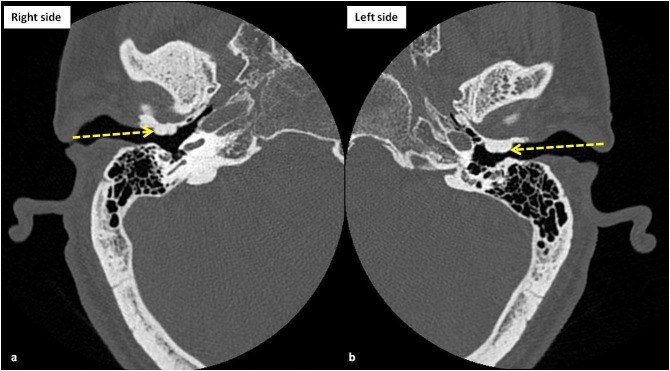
The computerized tomography (CT) scan images (bone windows) of right (a) and left (b) temporal bones at the level of external auditory canals show nearly symmetrical smooth bony outgrowths (yellow arrows), arising from the anterior walls of the external auditory canals, consistent with exostoses. Please note that the exostoses are causing significant narrowing of the external auditory canals leading to the patient’s symptoms of progressive conductive hearing loss, compatible with the diagnosis of surfer's ear.
